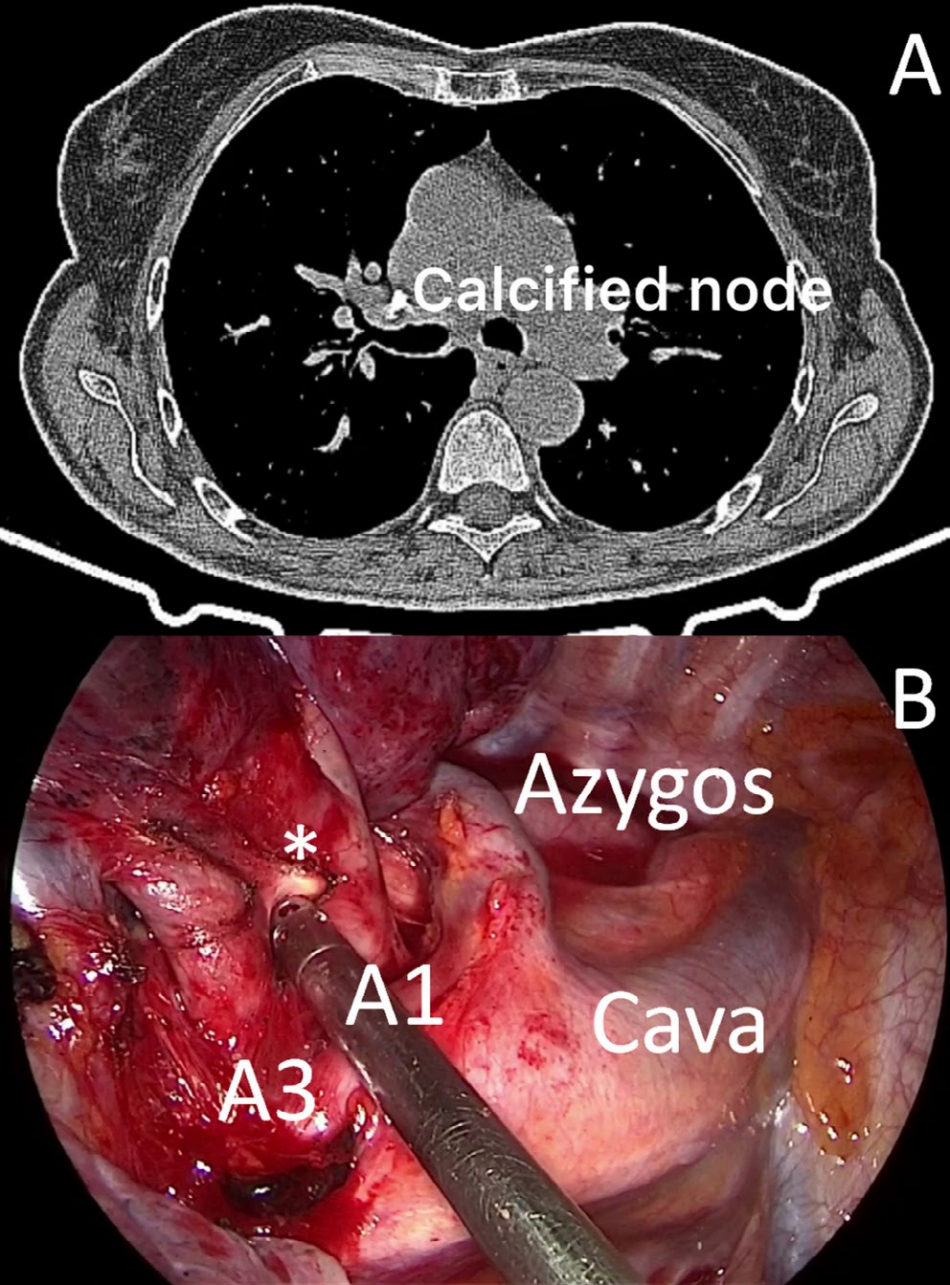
Chest CT scan (A) and intraoperative view (B) showed the presence of a calcified lymph node (*) between the A1 and the A3 branches of the mediastinal trunk of the pulmonary artery.
